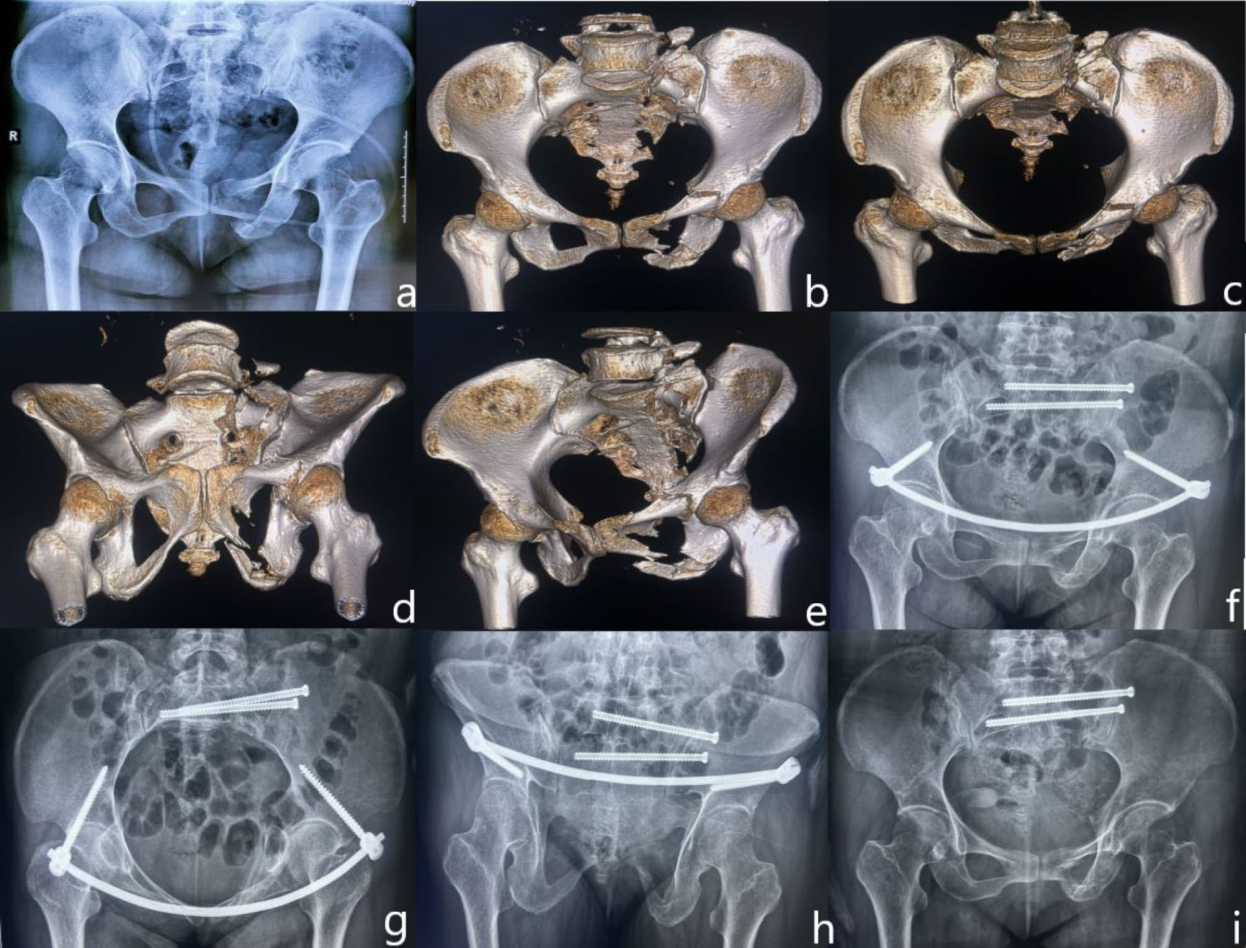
C-INFIX patient, female, 41 years old, preoperative diagnosis: OTA/AO 61B1.3. Posterior pelvic ring fixed by sacroiliac joint screws, anterior ring fixed with C-INFIX, titanium rod shaped according to Bikini area. (a) Preoperative anterior-posterior pelvic radiographs; (b) Preoperative 3D reconstructed anterior-posterior pelvic views; (c) 3D reconstructed pelvic inlet views; (d) 3D reconstructed pelvic outlet views; (e) Preoperative 3D reconstructed closed-hole oblique views; (f) Postoperative anterior-posterior pelvic radiographs; (g) Postoperative pelvic inlet radiographs; (h) Postoperative pelvic outlet radiographs; (i) Postoperative anterior-posterior pelvic radiographs after removal of the internal fixation
